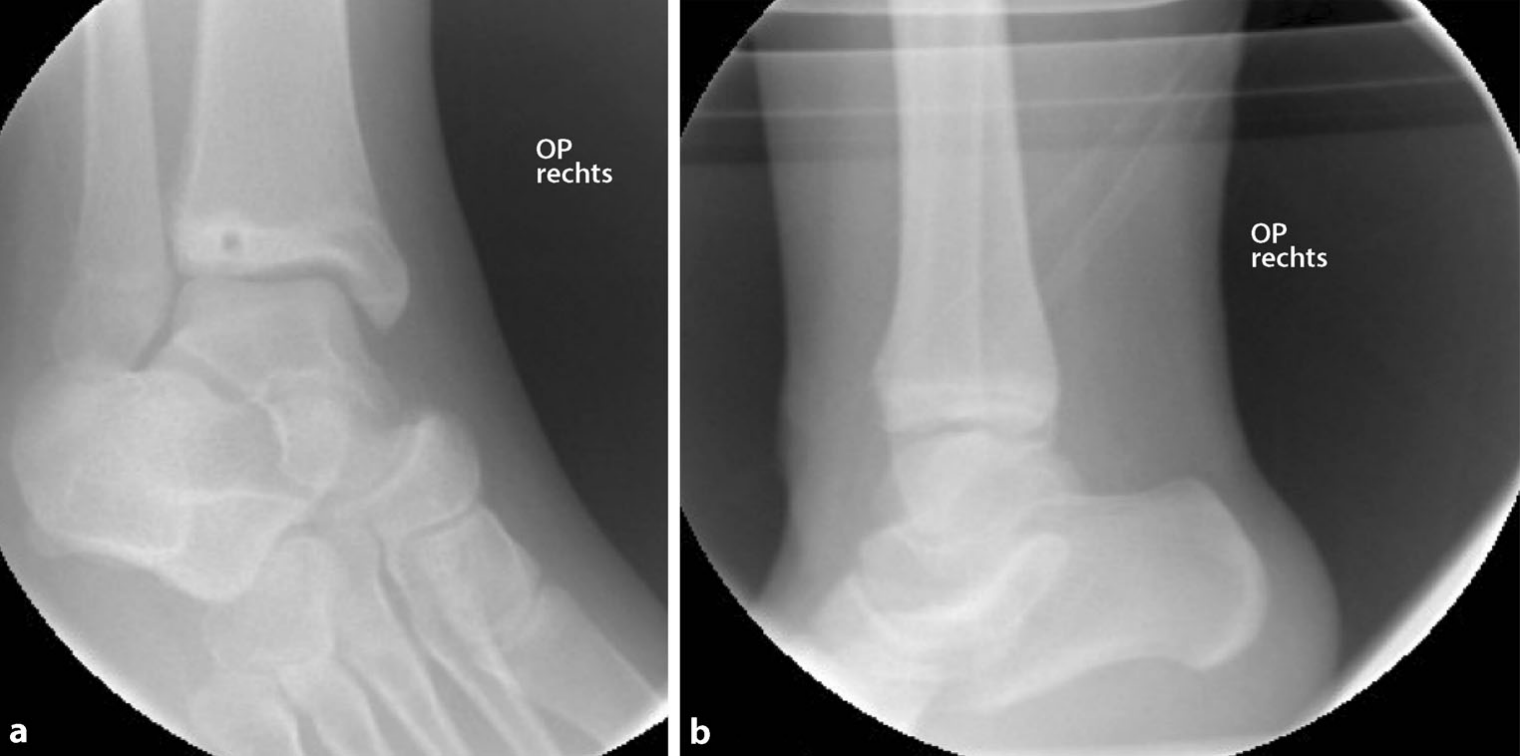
X-ray in two planes after screw removal in a 14-year-old patient (a anteroposterior view, b lateral view)
